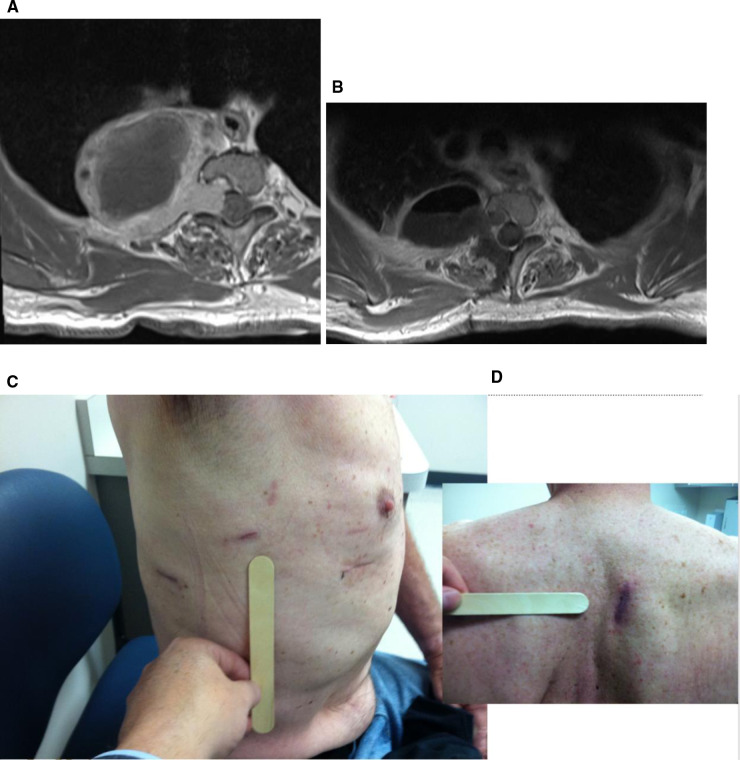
(A) Pre- and (B) post-operative axial MRI showing gross total tumor resection. Post-operative (C) anterior and (D) posterior thoracic incision after use of the da Vinci robot to remove apical thoracic tumor.
